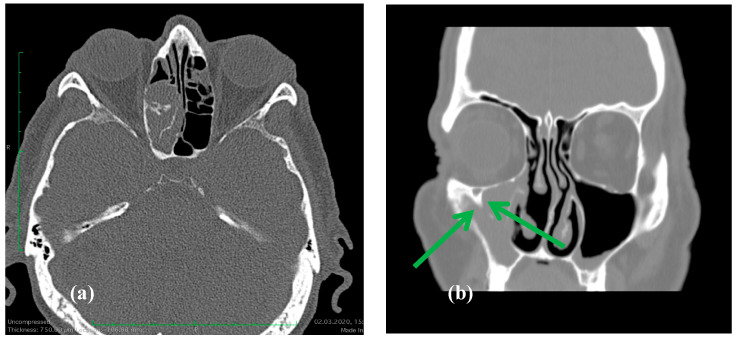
(a). Axial CT showing a right ethmoid sinus IP with focal hyperostosis along the lateral ethmoid wall, indicative of the tumor’s likely attachment site, a characteristic imaging feature of IP. (b). Coronal CT showing an inverted papilloma arising from the right maxillary sinus. Note the focal area of hyperostosis near the infraorbital canal (green arrows). The need to preserve the infraorbital nerve makes complete IP removal more difficult, increasing the risk of residual disease and recurrence.
